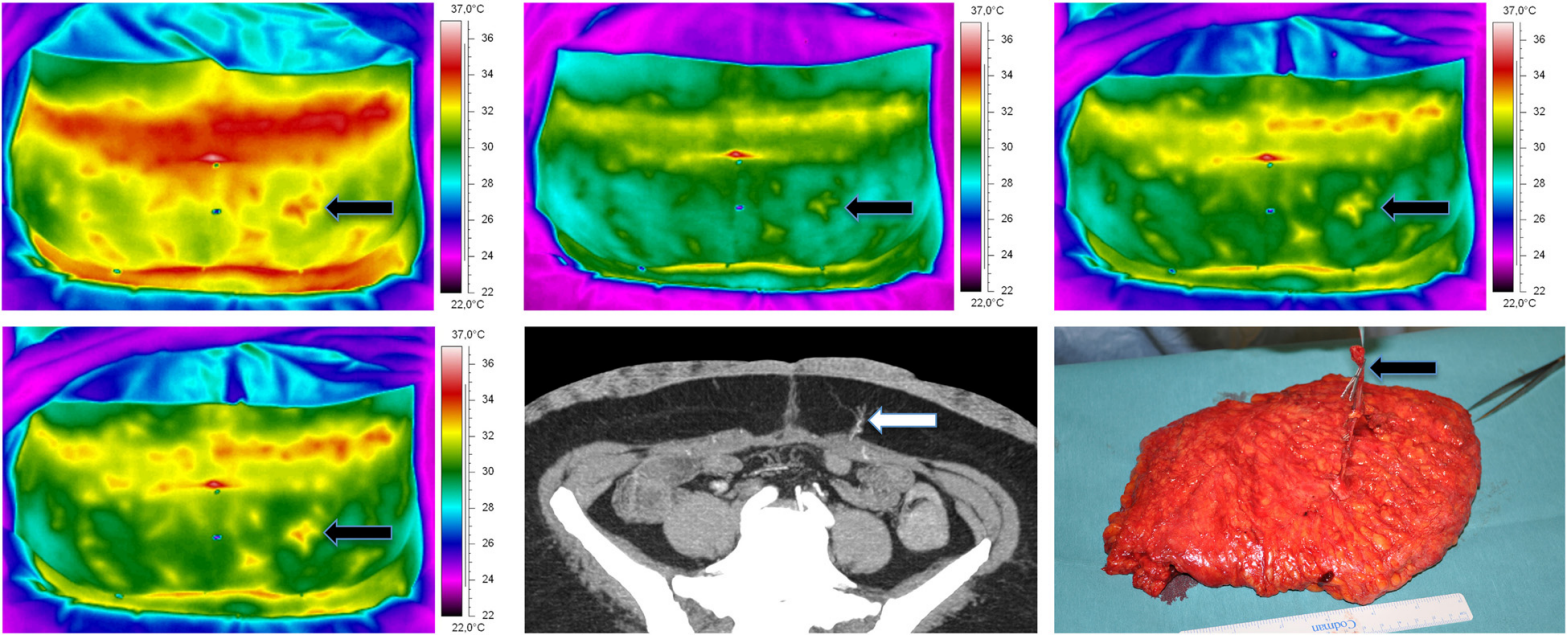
The selected hot spot (*arrow* on IR images) is associated with a perforator on CTA (arrow on CTA image) and intraoperatively (*arrow* on intraoperative flap image)
